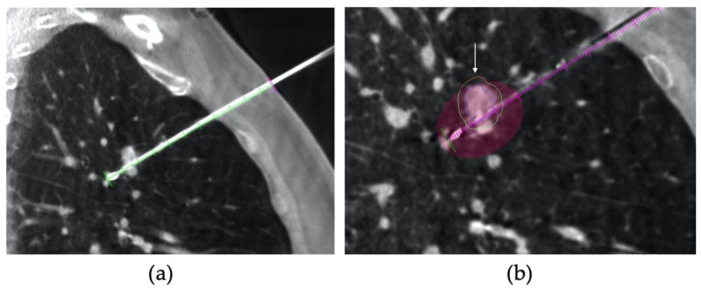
VPS was applied to CBCT images. (a) on sagittal CBCT scan, MWA is placed over the virtual one and (b) the predicted ablation volume is seen as a purple ellipsoid and the red line (white arrow) shows that the nodule is not completely encompassed in the predicted volume.
